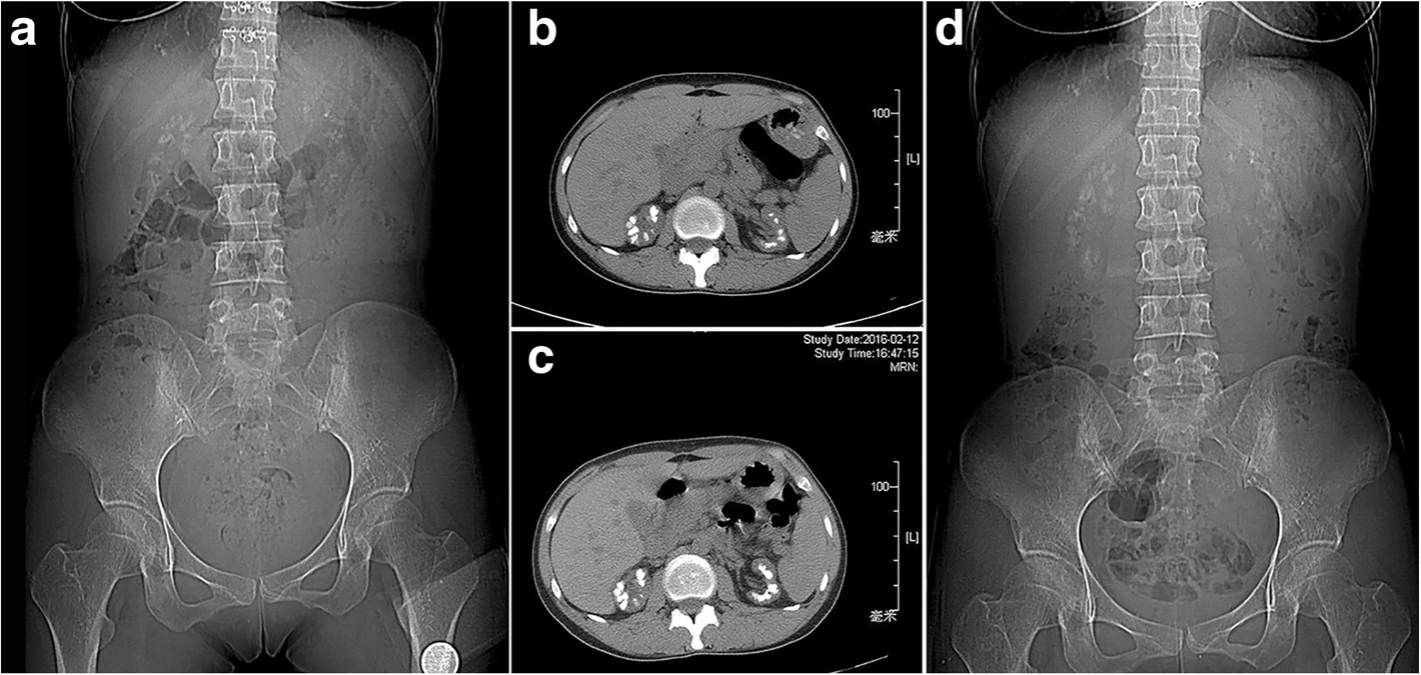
Imaging of renal tract in the proband. a. Plain abdominal radiograph showing bilateral nephrocalcinosis, aged 34 years. b. CT scan showing bilateral nephrocalcinosis, aged 34 years. c. CT scan showing bilateral nephrocalcinosis, aged 36 years. d. Plain abdominal radiograph showing bilateral nephrocalcinosis, aged 36 years
